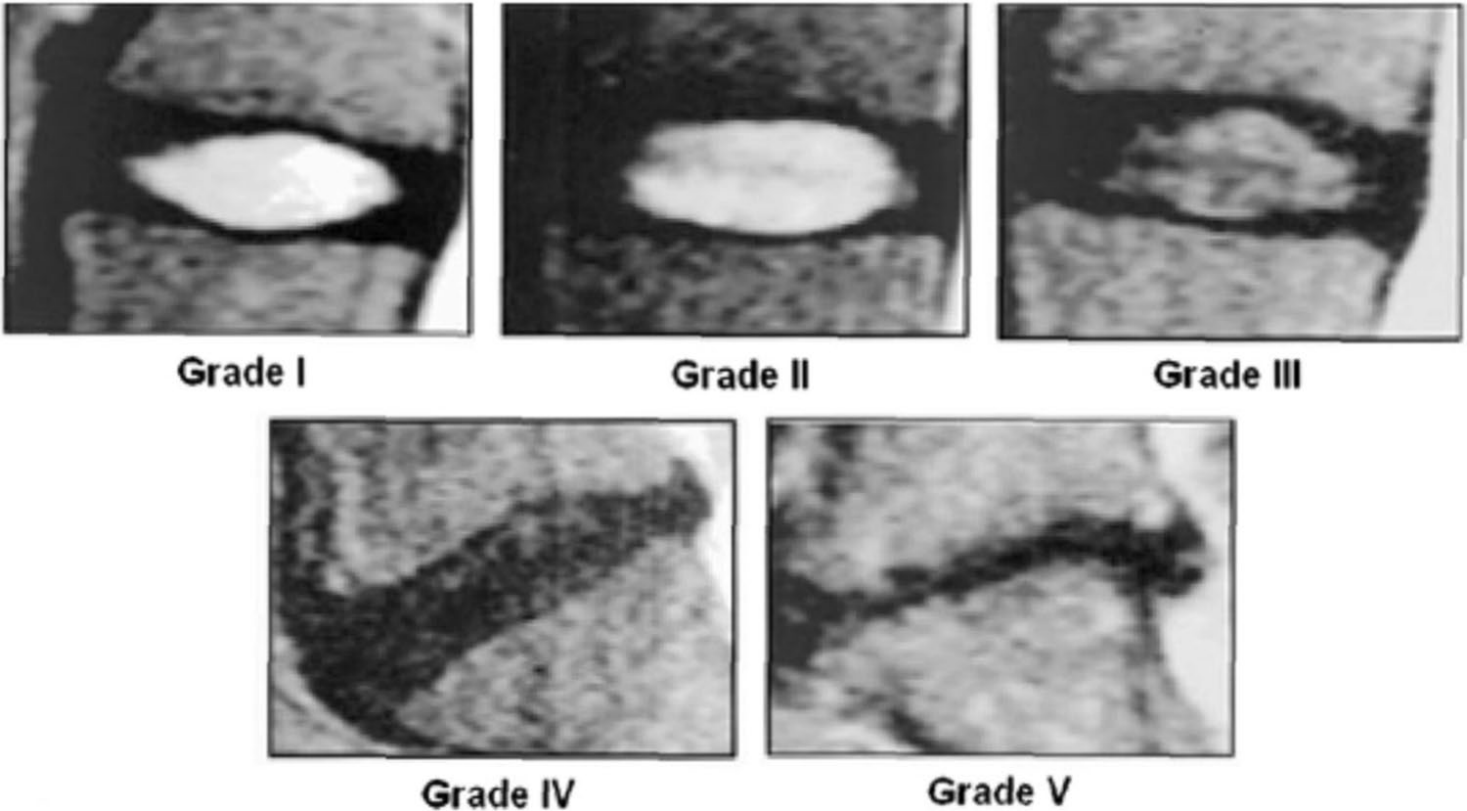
Pfirrmann grades on MRI. Image courtesy of Pfirrmann, C. W. A., Metzdorf, A., Zanetti, M., Hodler, J., & Boos, N. (2001). Magnetic resonance classification of lumbar intervertebral disc degeneration. Spine, 26(17), 1873–1878. 10.1097/00007632-200109010-00011
